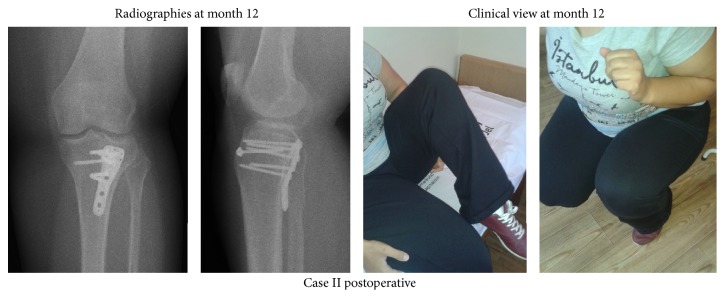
Postoperative 12th month radiographies and clinical view of Case II.
